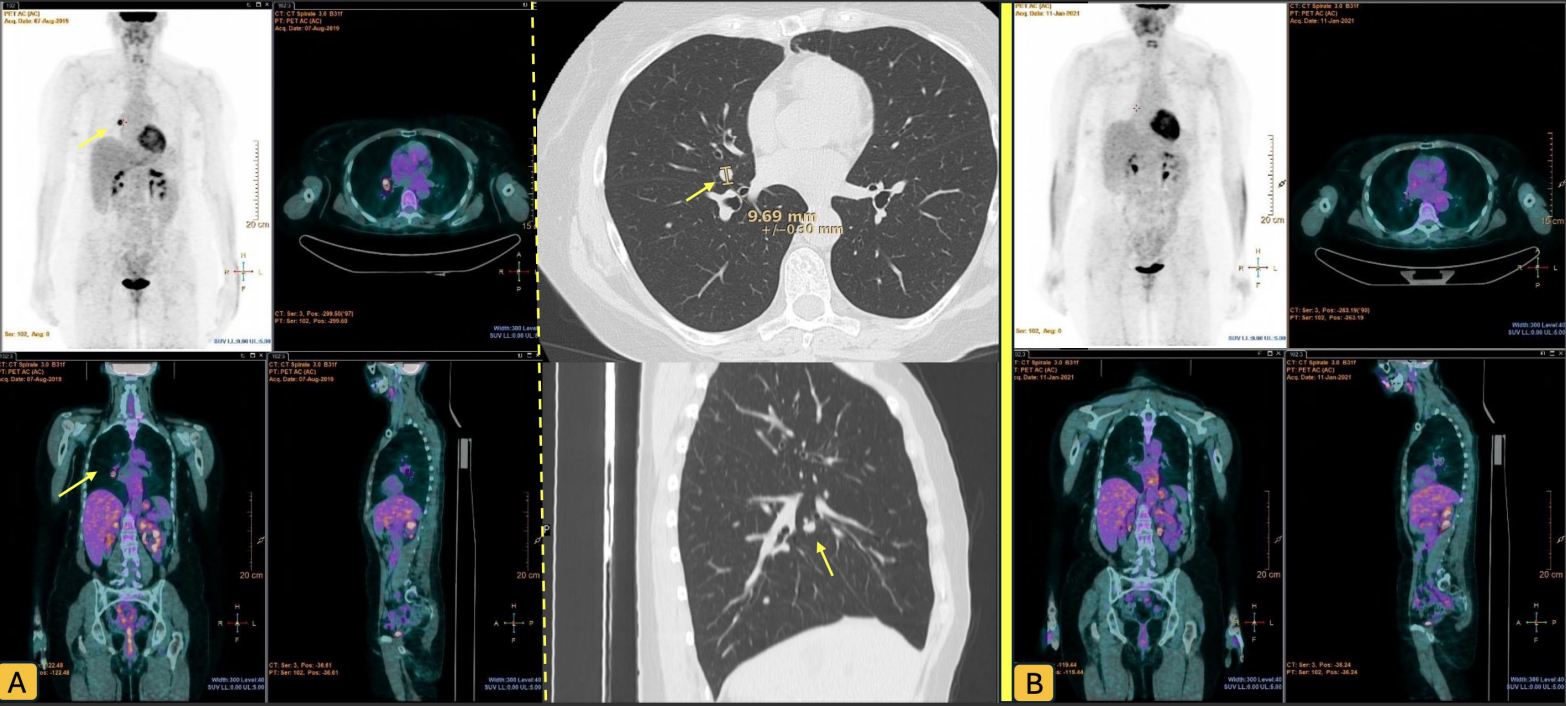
PET CT scan of patient 1 before (A) and after (B) lung lobectomy. Yellow arrows show the Pulmunary nodule.
